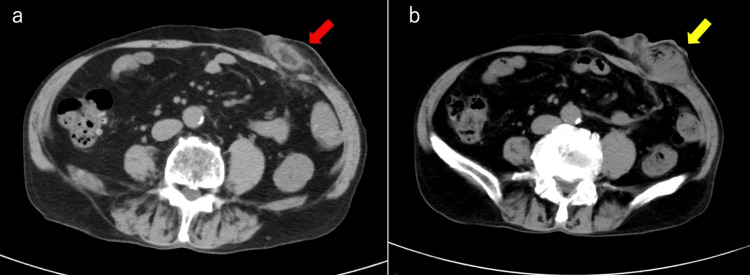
Abdominal CT findings. (a) Initial CT showing increased density in the subcutaneous fat surrounding the stoma (red arrow), consistent with cellulitis. (b) Repeat CT on the ninth day revealing gas and fluid collection (yellow arrow), indicating abscess formation.
